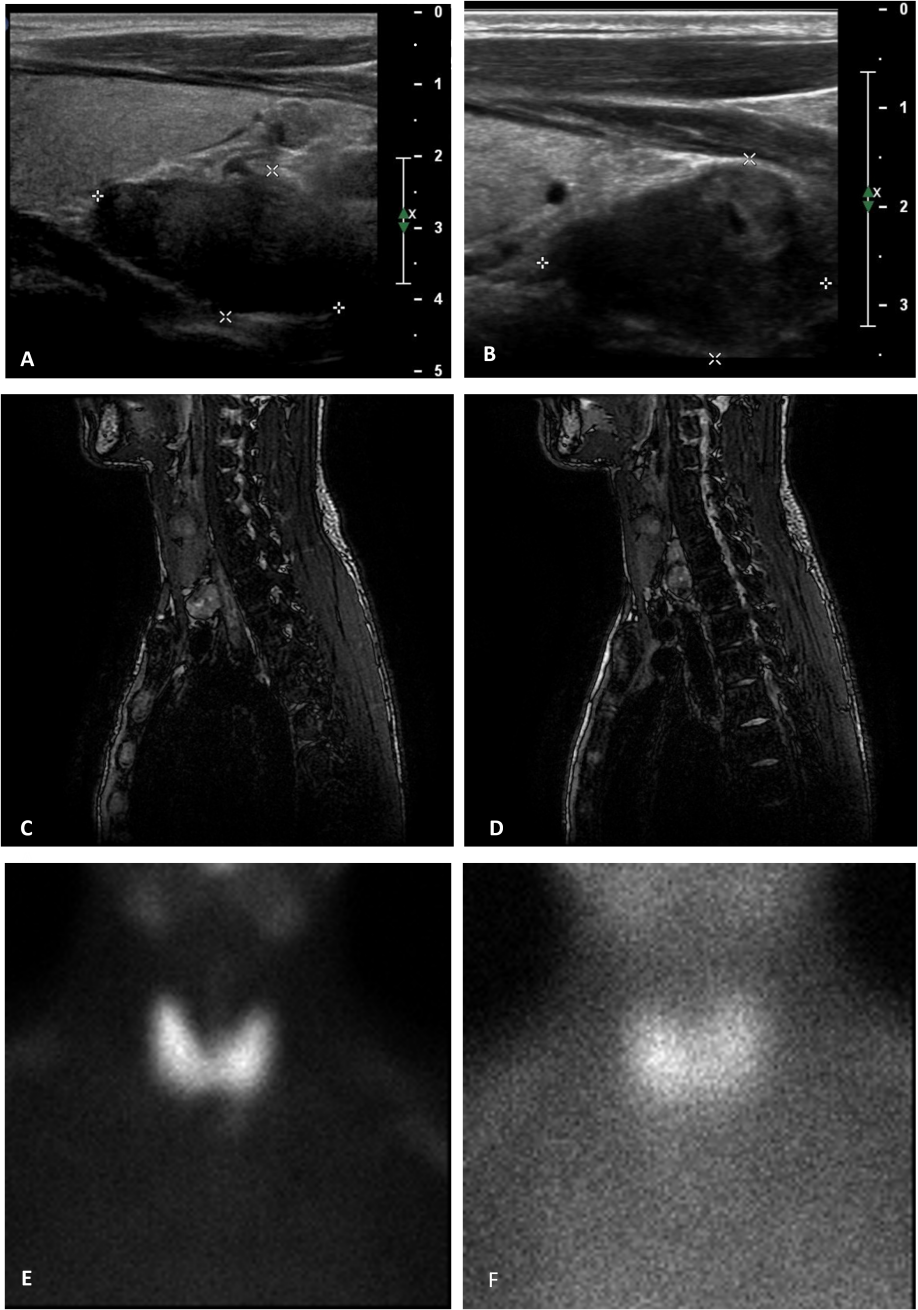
Summary of the patient's pre-operative examinations. (A, B) neck ultrasonography. Hyperplastic parathyroids are located below the level of the thyroid gland on the right (A) and left (B) sides of the neck (maximum diameter of 2.5 and 3.0 cm, respectively). (C, D) magnetic resonance Imaging. In the opposition phase, T2-weighted magnetic resonance images of the neck show hyperplastic parathyroid glands located under the left thyroid lobe (A) and behind the right thyroid lobe (B), characterized by hyperintensity signal, measuring 2.0 × 1.2 × 3.5 cm and 2.3 × 1.3 × 2.2 cm, respectively (APD × TD × LD). (E, F) parathyroid scintigraphy (99mTc 174 MBq + 99mTc-MIBI 326MBq). Pictures show an area of residual ^99m^Tc-MIBI fixation located near the left thyroid lobe, compatible with a hyperfunctional parathyroid.
